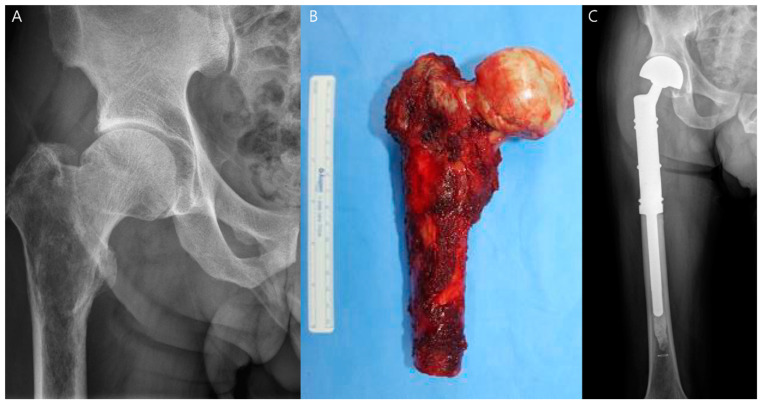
Tumor prosthesis at the proximal femur due to osteosarcoma of the proximal femur. (A) Preoperative plain radiograph shows an osseous tumor in the proximal femur, highly suggestive of osteosarcoma. (B) Gross clinical photograph of the widely excised tumor is shown. (C) Postoperative plain radiograph after endoprosthetic reconstruction of the proximal femur is shown.
